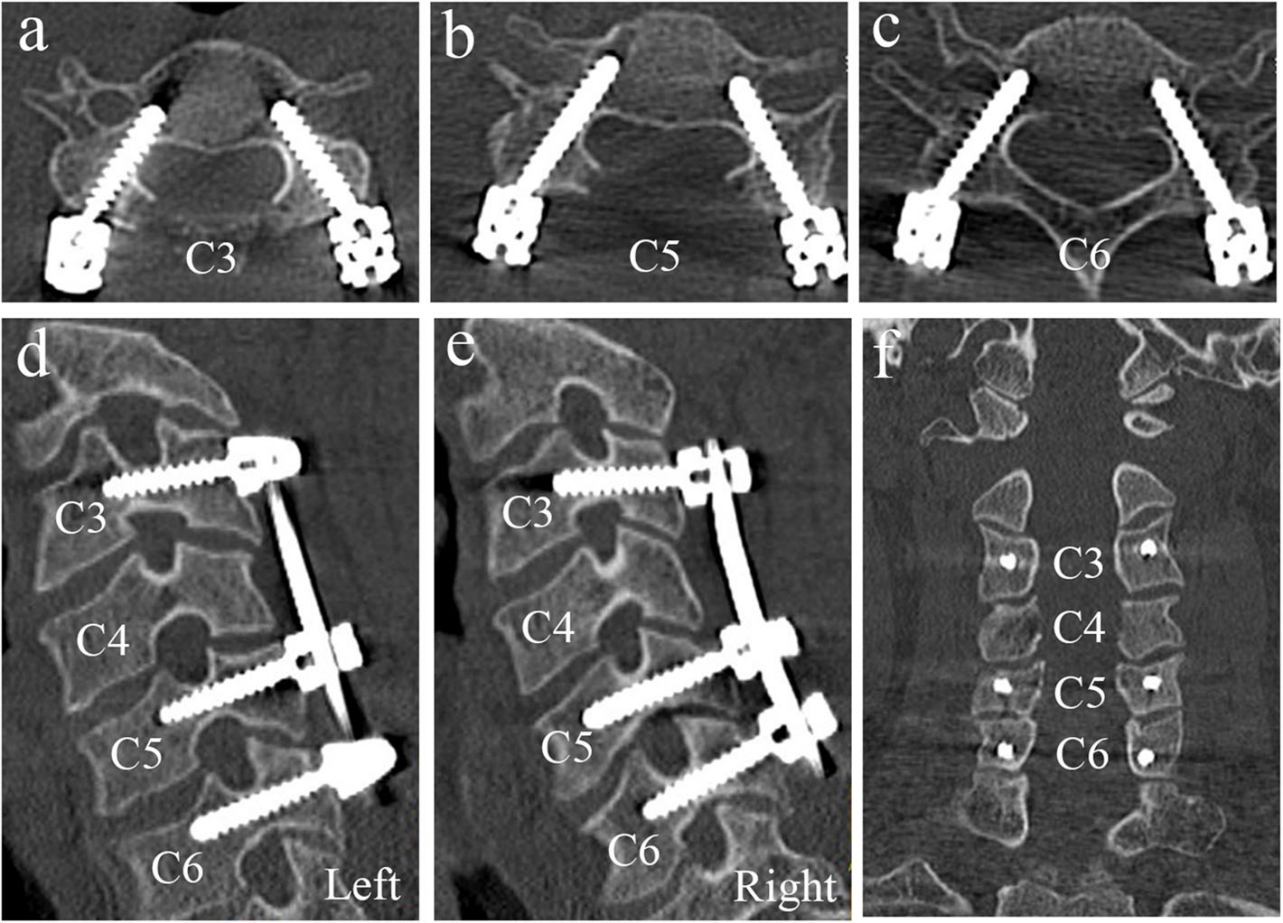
A typical case with successful cervical pedicle screw (CPS) fixation. Postoperative CT scans and multiplanar reconstruction showed the good placement of pedicle screws at C3, C5, C6 levels (a, b, c, d, e, f)
